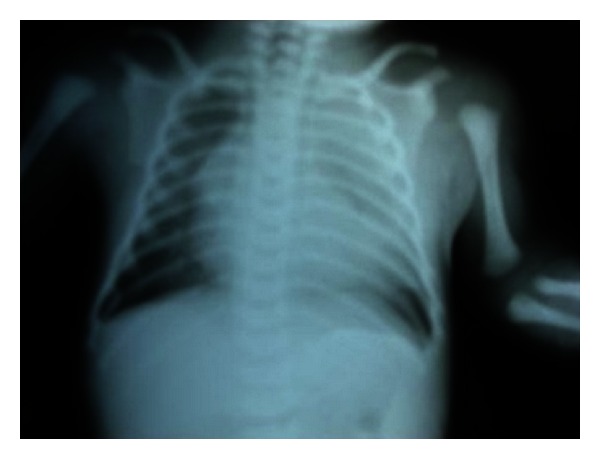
Postnatal chest X-ray in anteroposterior view showing the displacement of the heart and the mediastinum from the left to the right.
